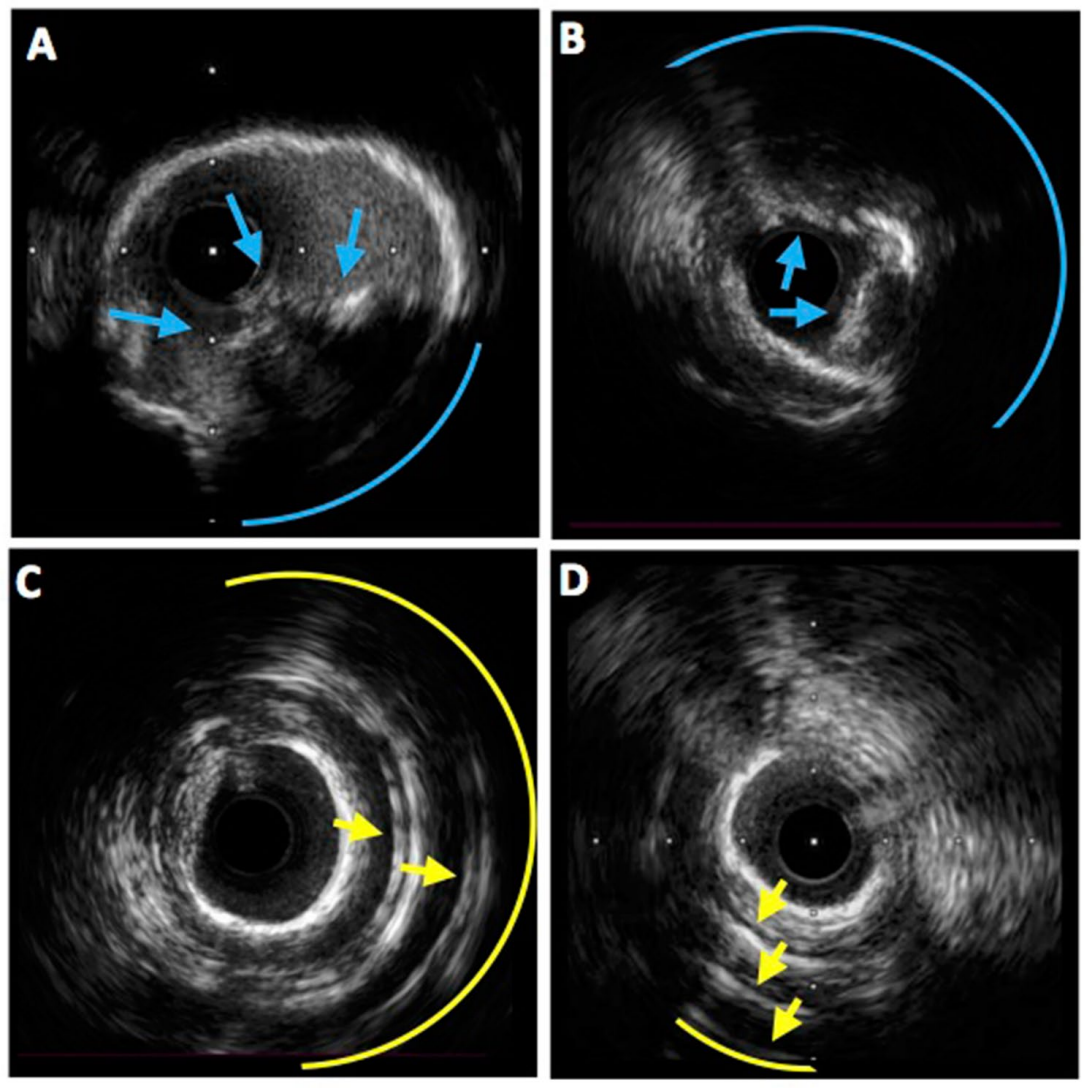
Evaluation of reverberation and calcified nodule. (A) Calcified nodule (blue arrows) showing irregular surface and convex shape with the distribution of one quadrant (blue arc). (B) A cross-sectional IVUS image showed calcified nodule (blue arrows) extending to two quadrants (blue arc). (C) A cross-sectional IVUS image showed two lines of reverberations (yellow arrows) with the distribution of nearly two quadrants (yellow arc). (D) There were three arctic lines of reverberations (yellow arrows) with the distribution of less than one quadrant (yellow arc). *IVUS* intravascular ultrasound.
